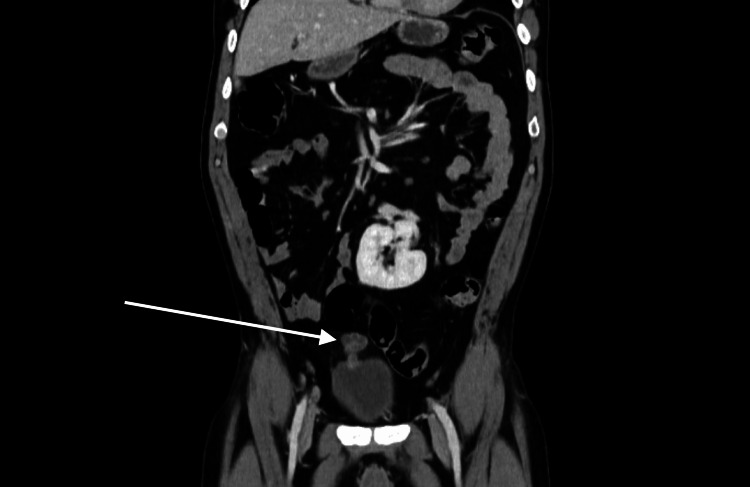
Contrast-enhanced CT of the abdomen showing a well-defined cystic lesion (arrow) at the anterosuperior aspect of the bladder with mild surrounding inflammatory changes.
